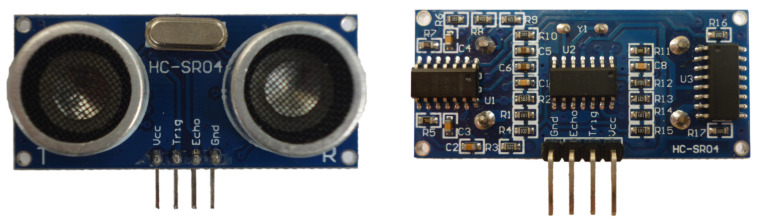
Picture of the HC-SR04 ultrasonic sensor. Top view in the left panel, bottom view in the right panel. The ultrasound emitter is labeled as “T” (left) and the receiver as “R” (right). It has 4 pins: “Vcc” and “Gnd” to provide the needed power, “Trig” to deliver the trigger signal that activates the ultrasound emission, and Echo”, which is activated when the echo is detected.
